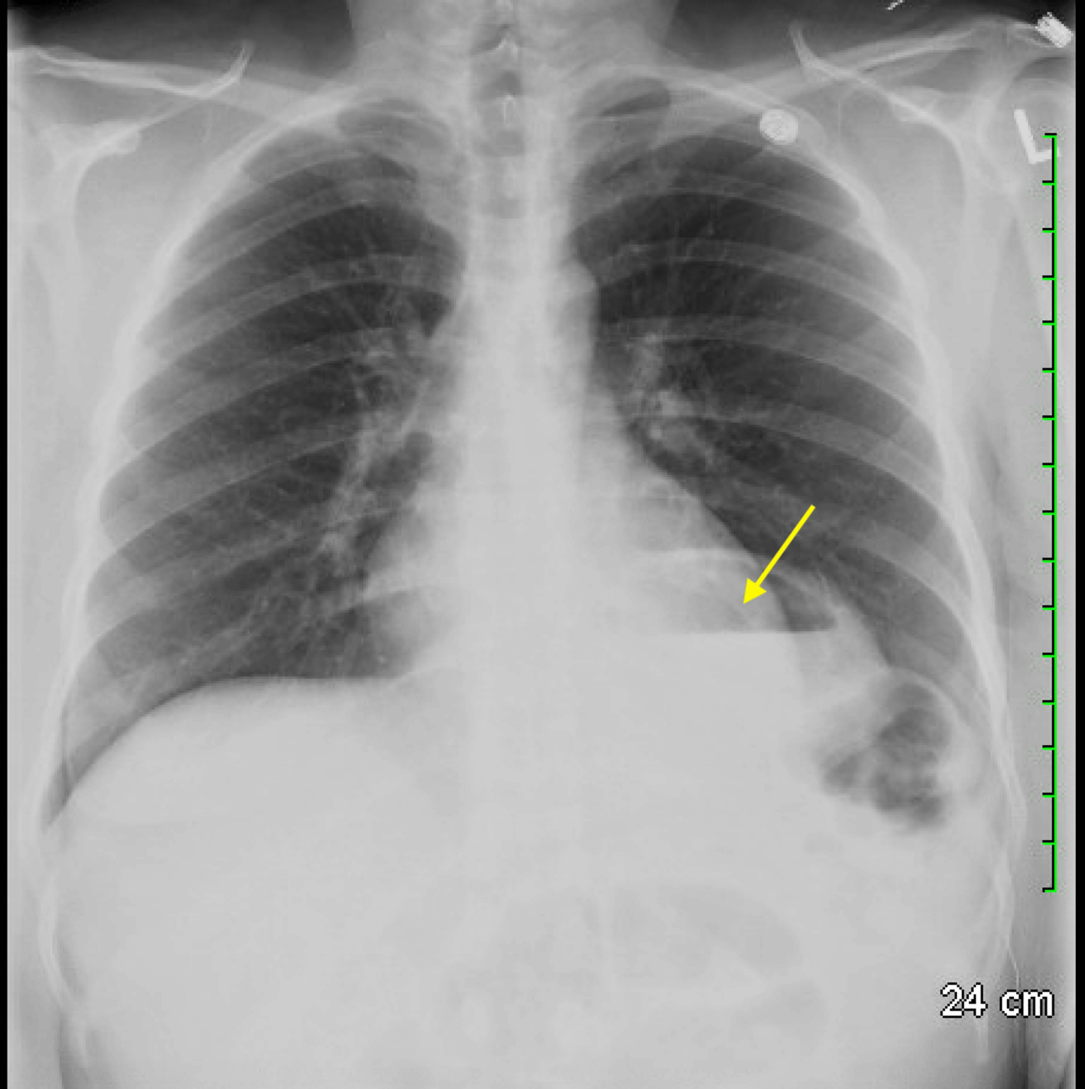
Chest X-ray showing a partial intrathoracic stomach (yellow arrow)
